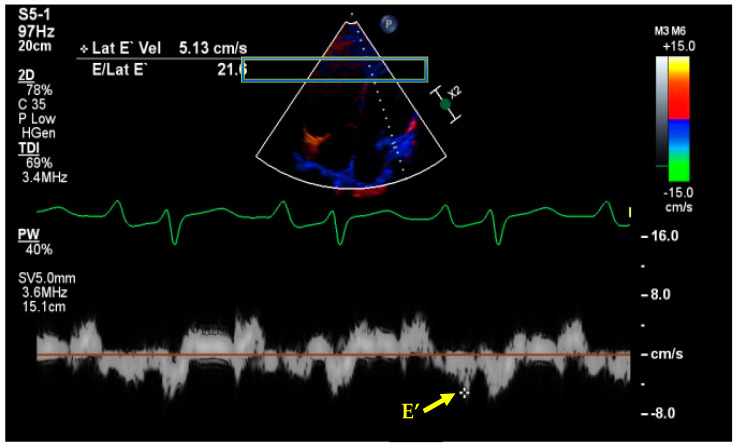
Tissue Doppler image (TDI) showing E’ and elevated E/E’ ratio implying elevated filling pressures. E wave calculated from Doppler analysis over MV inflow (not shown).
